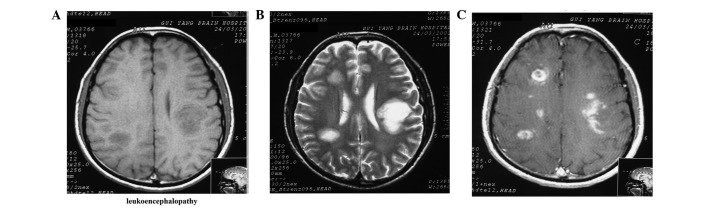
Case 1: (A) Axial T1-weighted imaging (T1WI) exhibits hypointense multiple foci at the bilateral peri-lateral ventricles and centrum ovale; (B) axial heavy T1-weighted imaging (T2WI) exhibits hyperintense foci; and (C) axial enhancement demonstrates patch-, ring- and ring cleavage-like foci.
